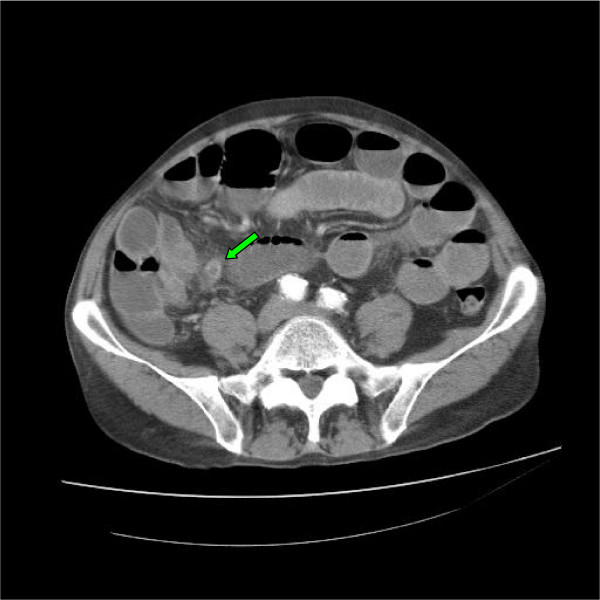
Abdominal CT image of patient 1. This image shows the presence of an inflammatory process in the right iliac fossa (green arrow).
